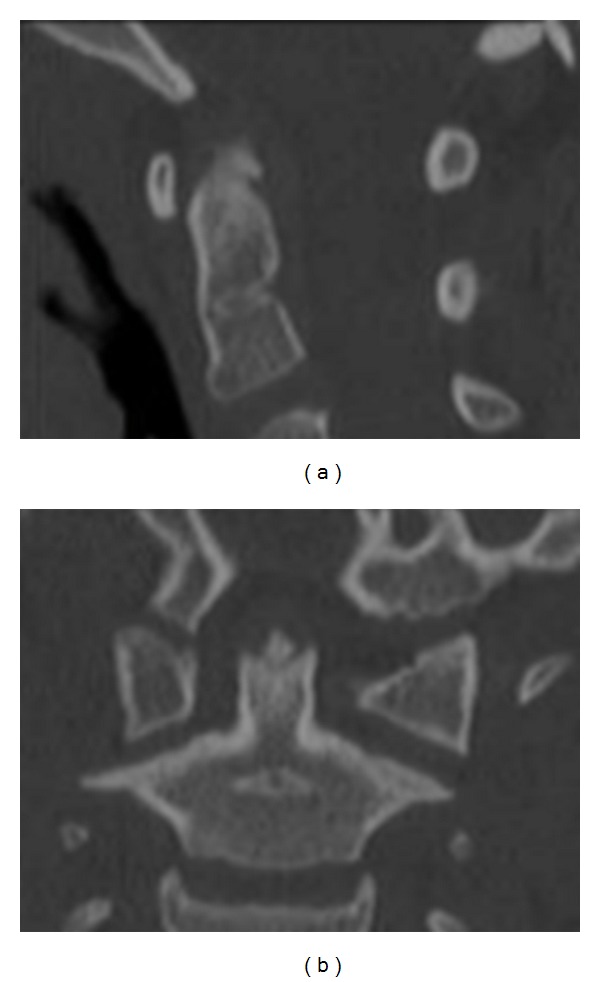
(a) Sagittal and (b) coronal reformatted CT scan confirming normal appearance of the odontoid peg.
